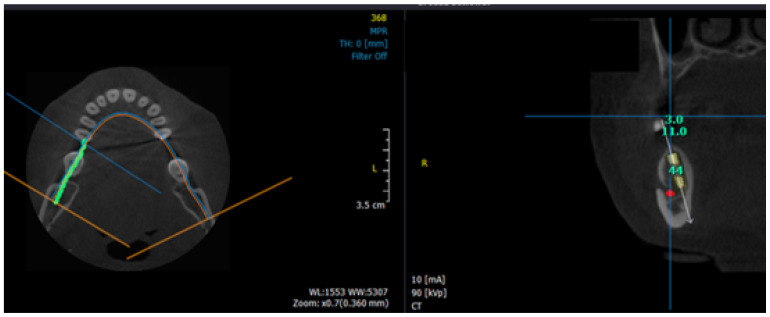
Inferior alveolar nerve tracing on axial plane view of CBCT and virtual implant placement by bypassing inferior alveolar nerve in cross-sectional view.
